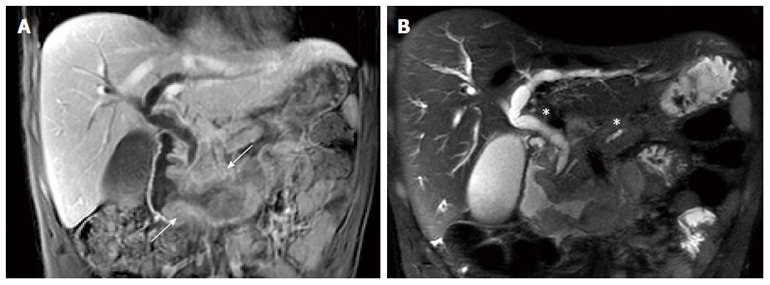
Magnetic resonance imaging. A: MRI, coronal T1-weighted MR image with contrast showing the duodenal tissue thickening spreading from the second duodenum proximal part up to the duodenojejunal flexure (white arrow); B: MRI, coronal T2-weighted MR image showing the pancreatic duct and bile duct upstream swelling (white asterisks) without any secondary hepatic lesion. MRI: Magnetic resonance imaging.
